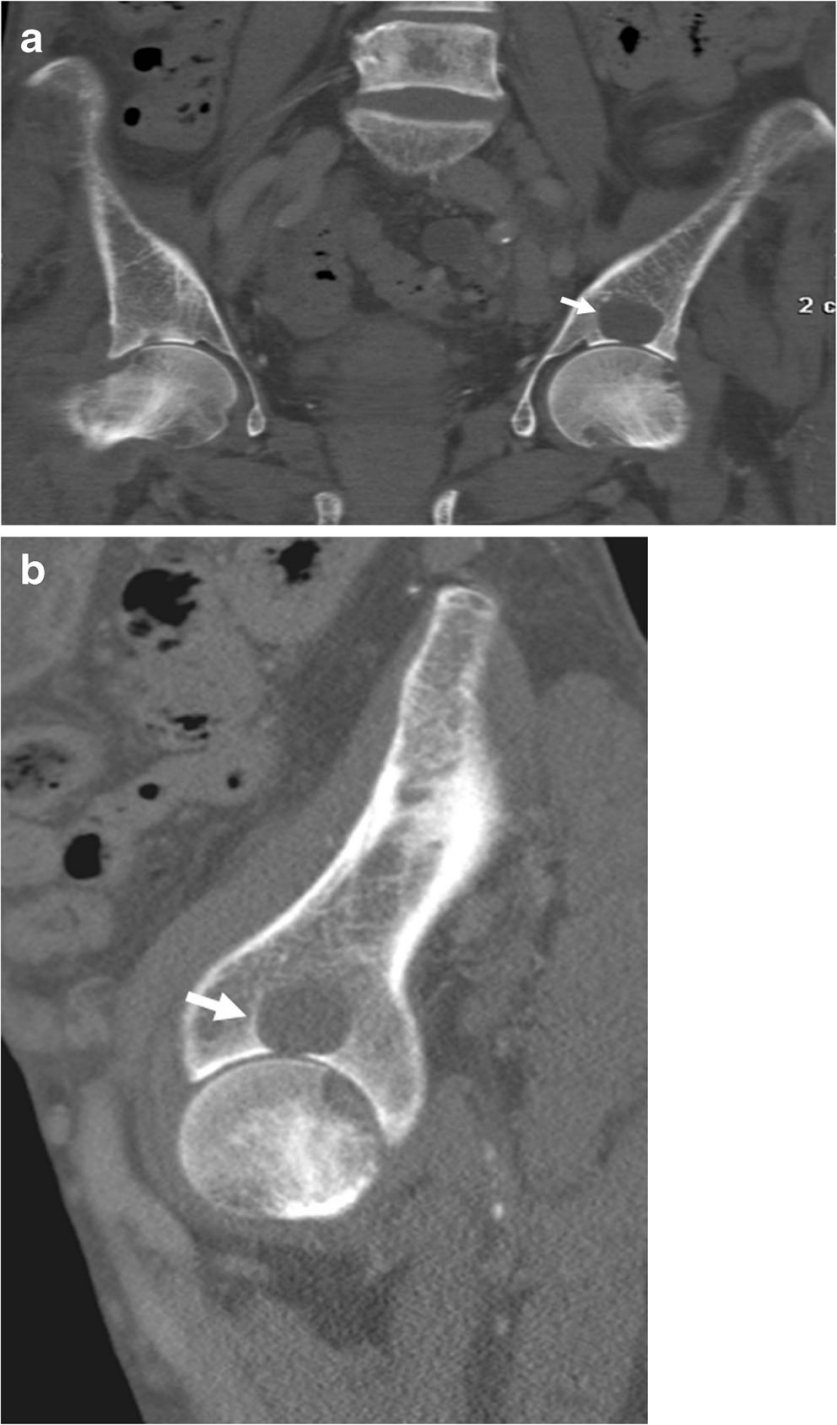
a, b: Unenhanced CT, coronal (a) and sagittal (b) reformations. Brown tumour in a patient undergoing chronic haemodialysis with tertiary hyperparathyroidism. The brown tumour appears as a lytic lesion of the ilium (*arrow*)
